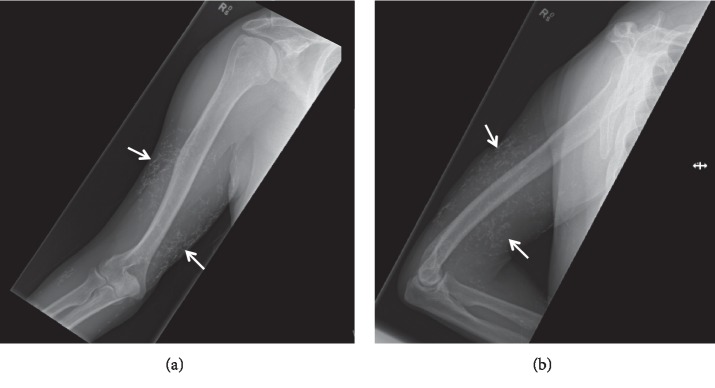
Radiographs of the anterior (a) and posterior (b) right arm and forearm show extensive linear and irregular calcifications (arrows) in the field of view. The distribution of the calcified foci includes the subcutaneous fat.
